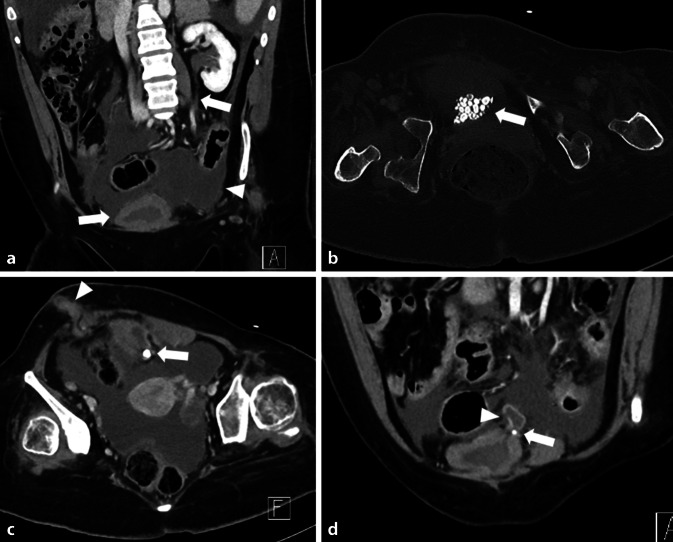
Computertomographie (CT) des Abdomens mit Kontrastmittel in portalvenöser Phase in koronarer (a, d) und axialer (b, c) Rekonstruktion (a, c, d Weichteilfenster, b Knochenfenster). a Es zeigt sich im Unterbauch die zirkulär wandverdickte Harnblase und der kontrastmittelaufnehmende Ureter links (*Pfeile*). Zudem ist reichlich Flüssigkeit im Unterbauch und ein verdicktes, kontrastmittelaufnehmendes Peritoneum zu erkennen (*Pfeilspitze*). b Innerhalb der Blase finden sich multiple röntgendichte Konkremente (*Pfeil*). c Die Blase zeigt ein nach links kranial gerichtetes Divertikel mit einem darin befindlichen Konkrement (*Pfeil*). Die Ausleitung des Mitrofanoff-Stoma im rechten Unterbauch ist verdickt und nimmt Kontrastmittel auf (*Pfeilspitze*). d Abgebildet ist erneut die wandverdickte Blase mit dem nach links kranial gerichteten Divertikel und dem teilweise erfassten Konkrement (*Pfeil*). Die Wand des Divertikels zeigt eine Konturunterbrechung (*Pfeilspitze*)
